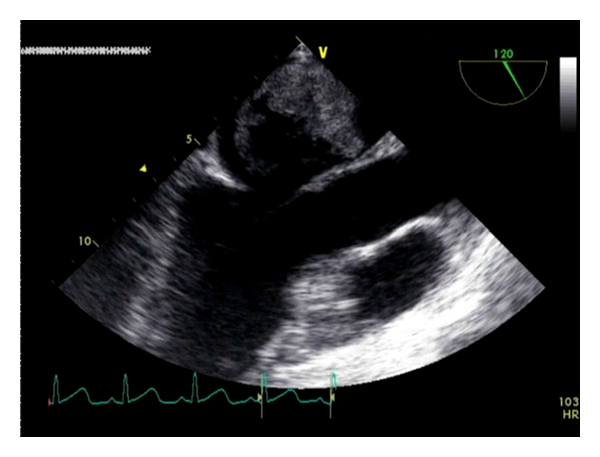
Transesophageal echocardiography showing left atrial myxoma.
